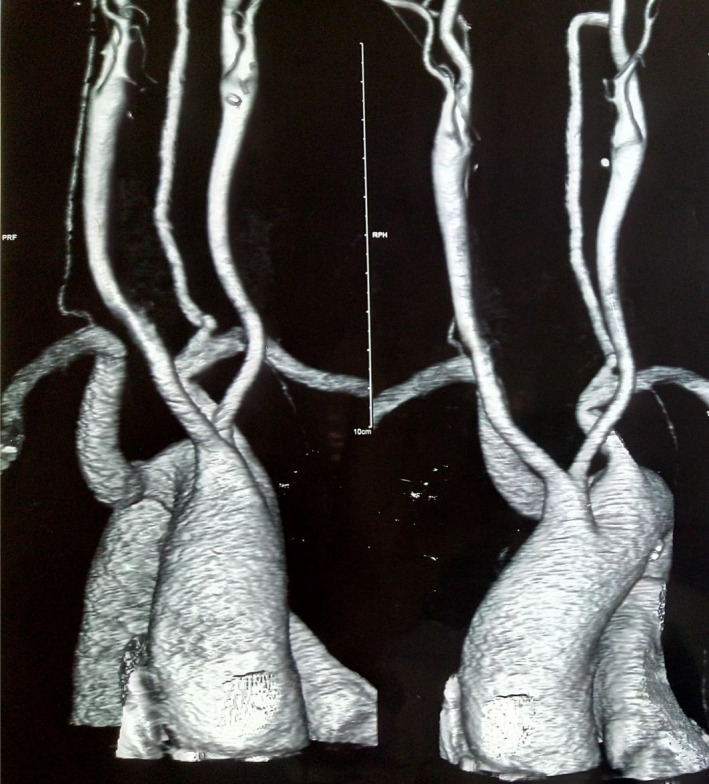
3D reconstruction of contrast‐enhanced CT scan showing an aberrant subclavian artery (lusoria)
